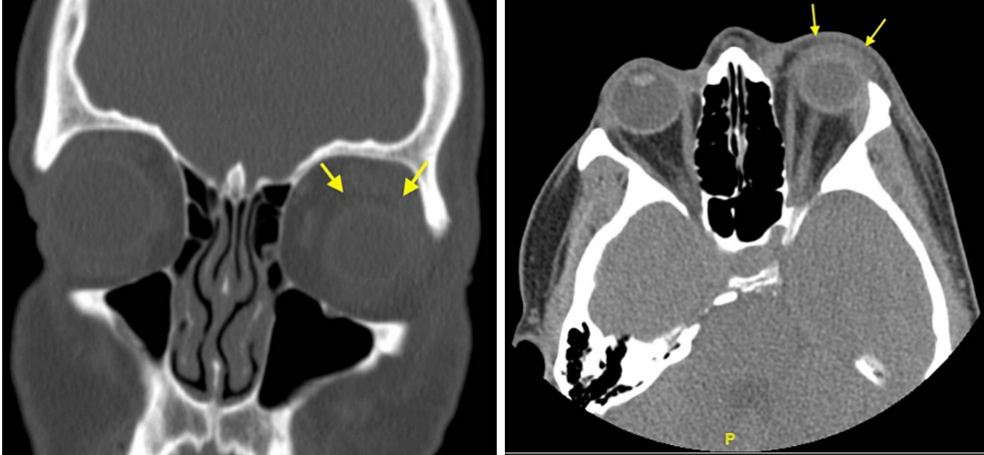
Coronal and axial CT views of the orbits; the arrows denote periorbital edema and soft tissue swelling.
